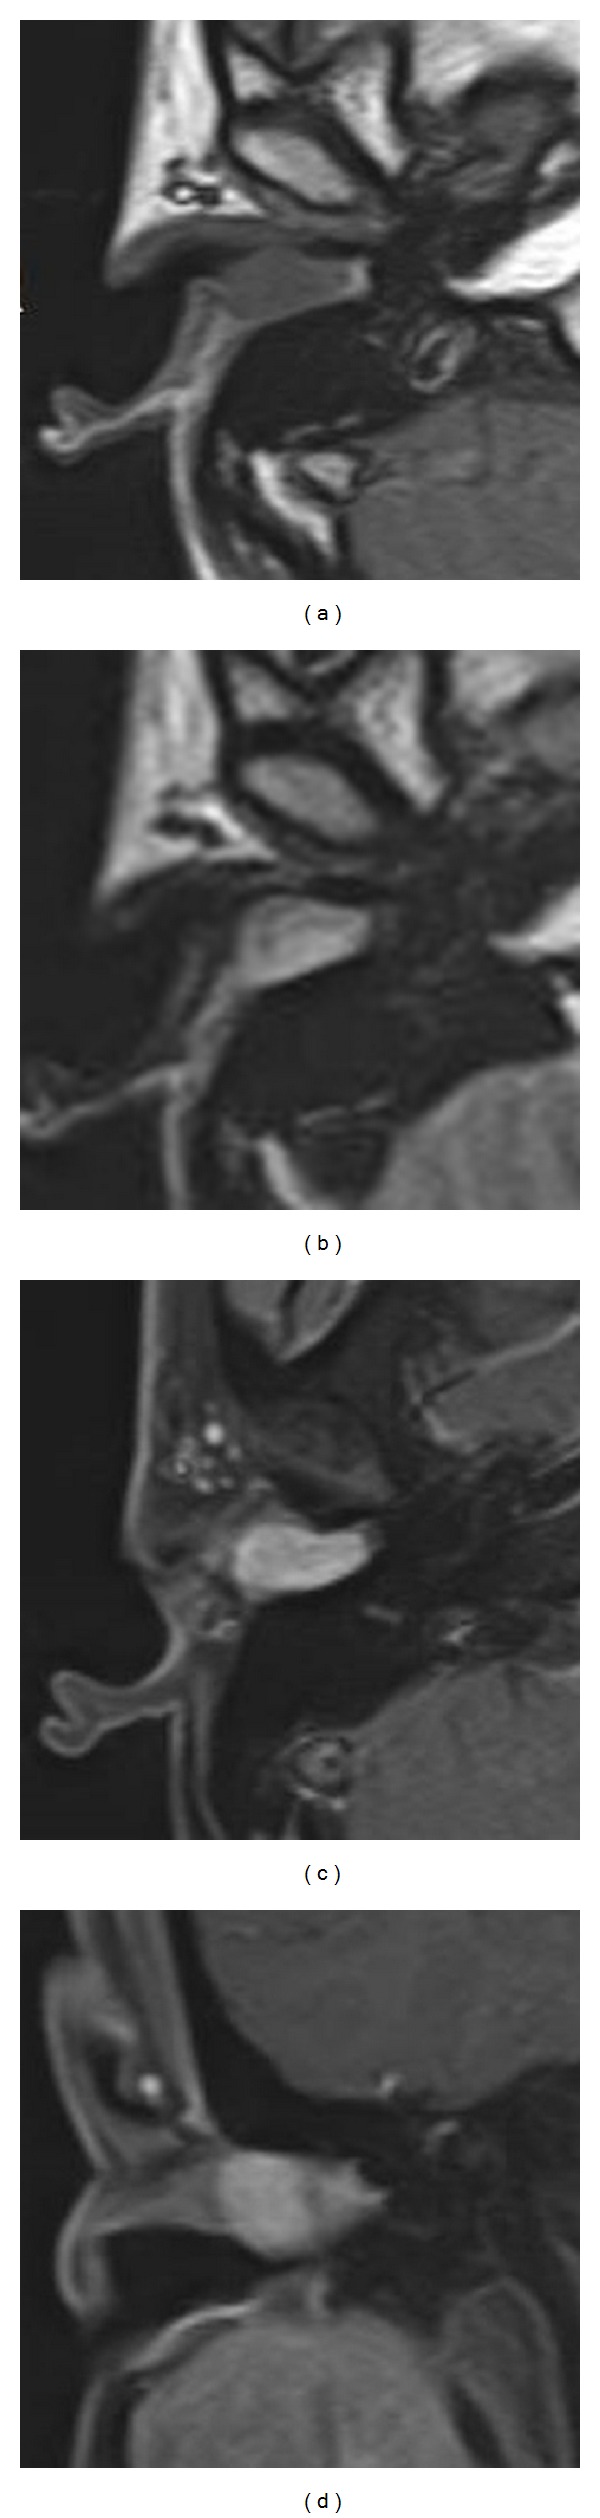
Magnetic resonance images. The tumour was detected as a 2.3 × 2.1 × 1.8 mm mass in the EAC, with low signal intensity on T1-weighted, high signal intensity on T2-weighted, and homogeneous enhancement on gadolinium-enhanced T1-weighted images. (a) Transverse section of a T1-weighted image. (b) Transverse section of a T2-weighted image. (c) Transverse section of a gadolinium-enhanced T1-weighted image. (d) Coronal section of a gadolinium-enhanced T1-weighted image.
